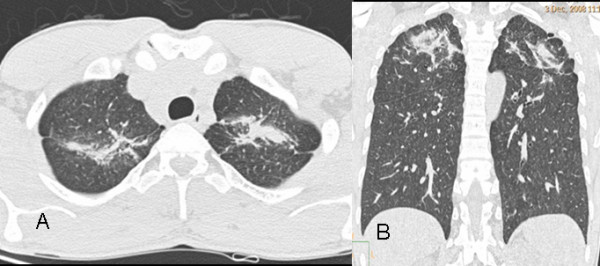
a, b - PMF and bands leading to parenchymal distortion in adjacent regions in both upper lobes of the lung are apparent on axial (a) and coronal (b) CT sections of a 27-year-old man who worked as a denim sandblaster for 48 months.
